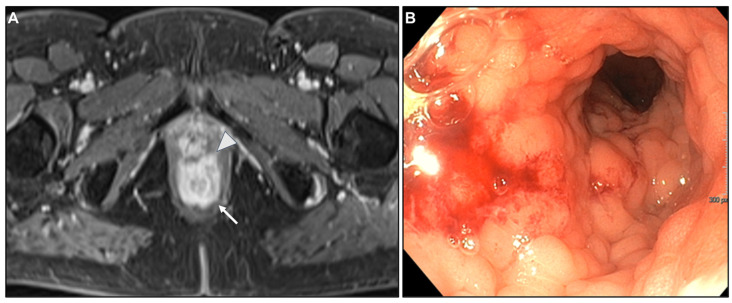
Infiltration of the rectovaginal septum. (A) MR image: Axial CE-GRE-T1WI. Linitis plastica-like rectal metastases (arrow) from breast adenocarcinoma with infiltration of the rectovaginal septum (arrowhead). (B) Corresponding colonoscopy reveals an extrinsic stenosis of the anus and rectum with rigid walls.
